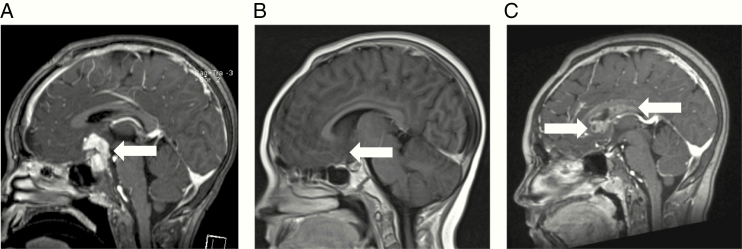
MRI of Case 4 with germinoma relapse of an intracranial malignant non-germinomatous germ cell tumor (NGGCT). Representative sagittal views at (A) original diagnosis of the NGGCT, (B) following completion of treatment with chemotherapy and radiotherapy, and (C) at the time of relapse with germinoma, with nodular enhancing cyst-like lesions posterior and inferior to the corpus callosum and involving the septum pellucidum. White arrows indicate the radiological abnormality.
